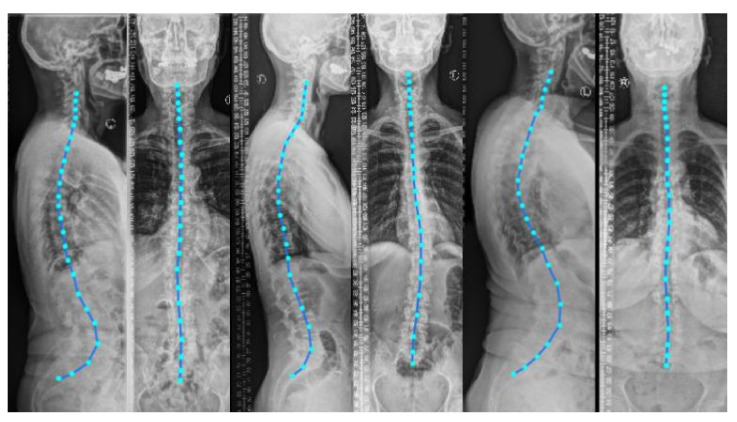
Lateral and anterior-posterior (AP) view radiographs of three patients with spinal curvature annotation. Considering the vertebral centroid coordinates and spinal curvature facilitates determining the scale and the vertebral orientation.
